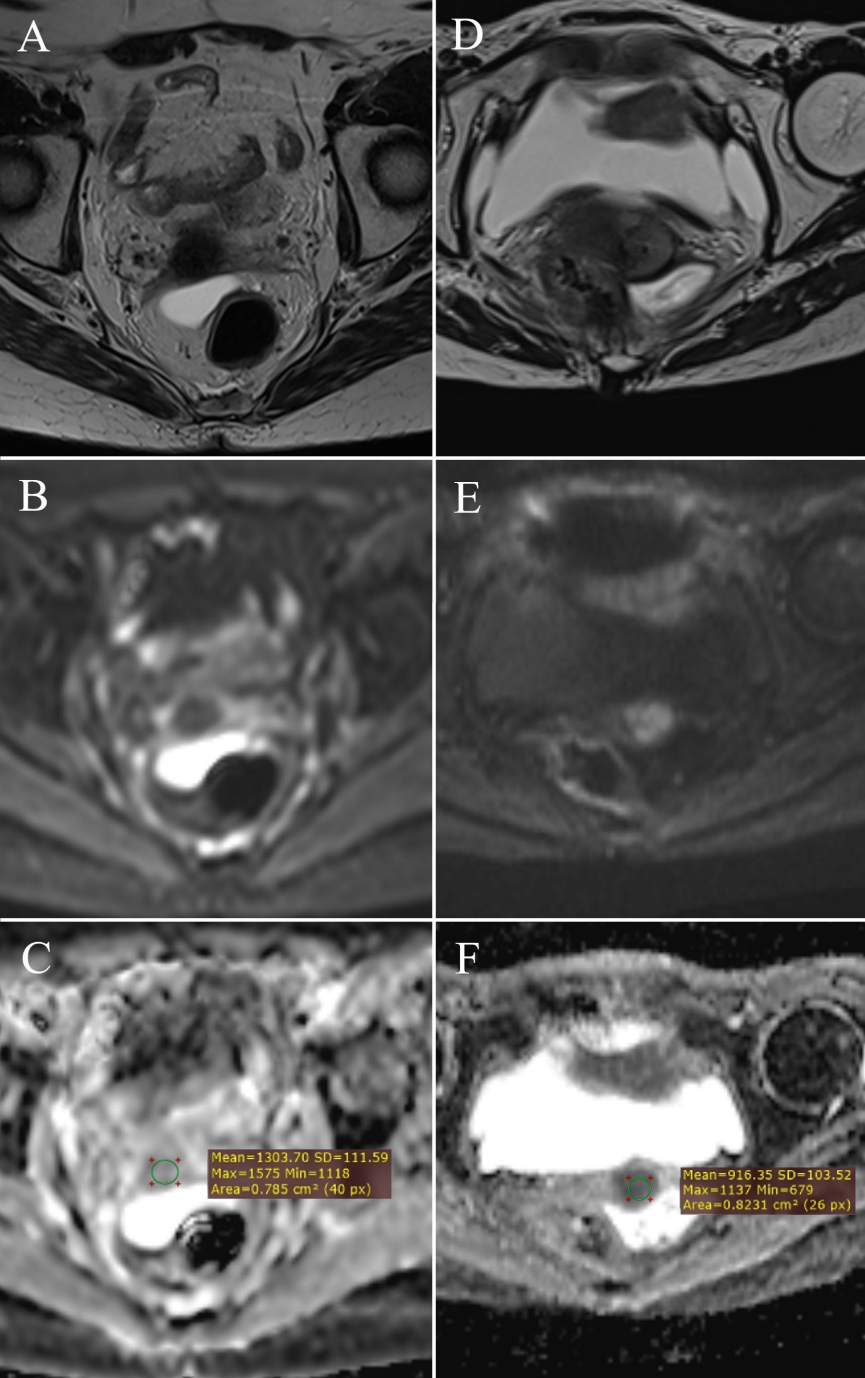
ADC value measurement: post-therapy cervix without tumor residue – (A) (T2W), (B) (DWI), (C) (ADC map with ROI); post-therapy cervix with tumor residue – (D) (T2W), (E) (DWI), (F) (ADC map with ROI).
